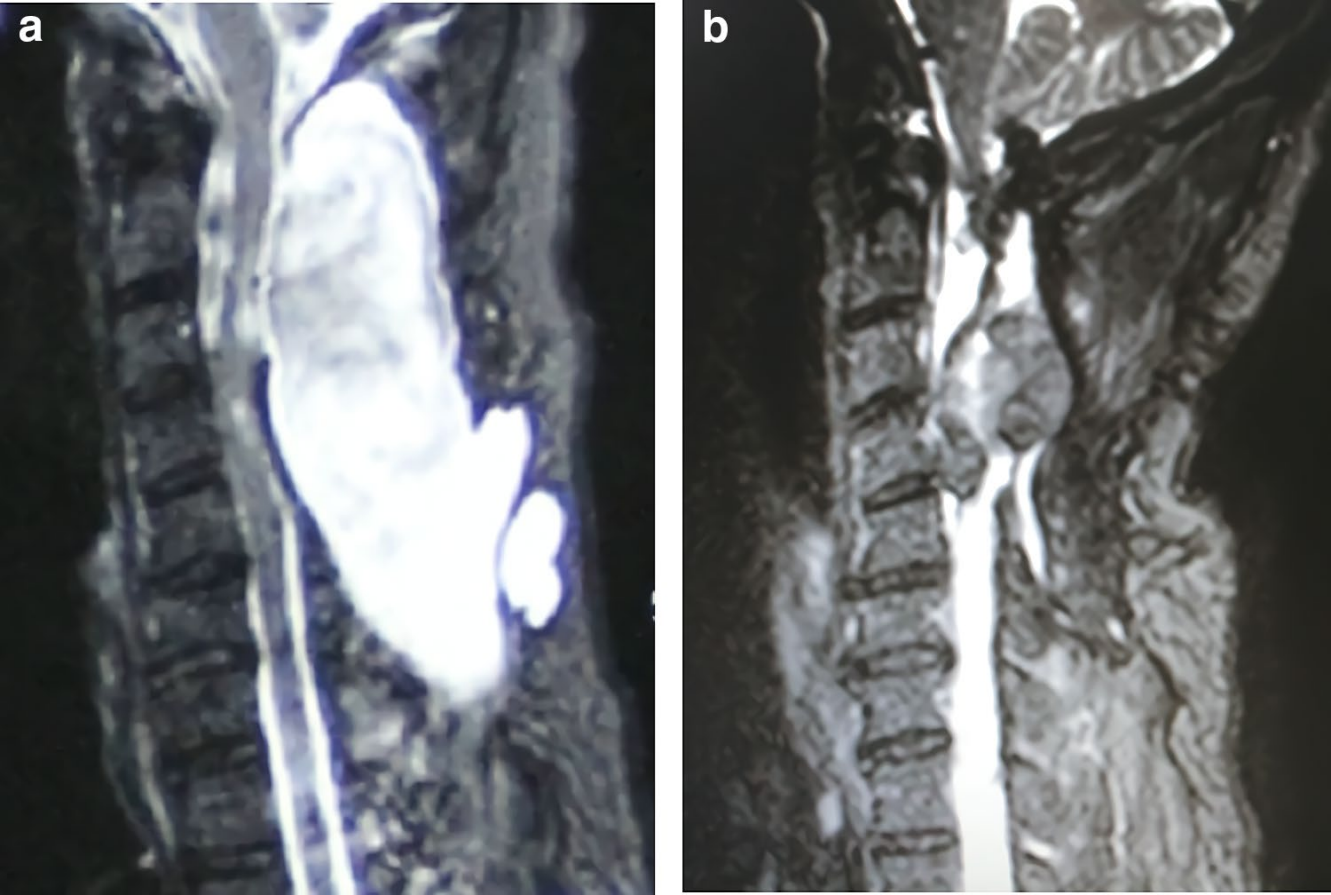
The first case. (a) Pre-operative area of the pseudomeningocele on sagittal MRI section. (b) Post-operative area of the pseudomeningocele on sagittal MRI section.
